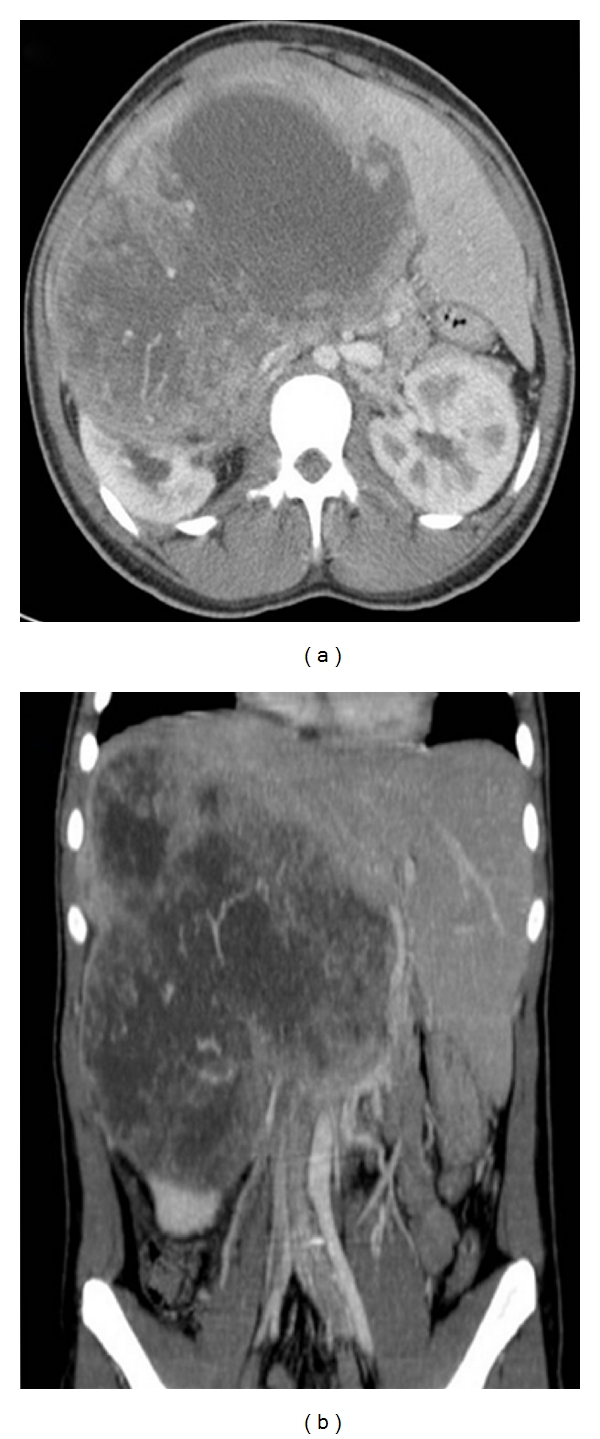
Preoperative CT scan showing a large, solid mass in the liver.
